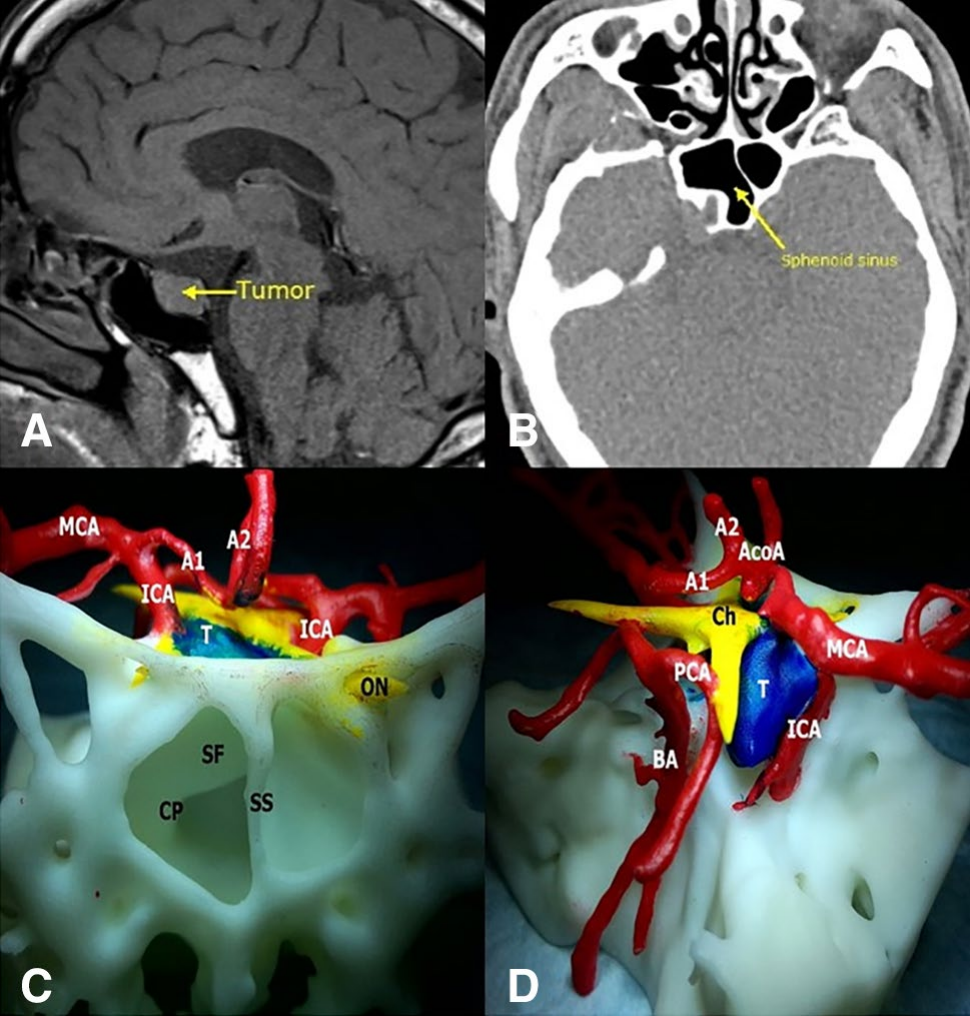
a MRI of the sella turcica region showing tumor lesions. The yellow arrow points to the tumor. b CT of the sphenoidal sinus. The yellow arrow points to the sphenoidal sinus. c, d Frontal and lateral views. *SF* sellar floor, *SS* sphenoid septation, *CP* carotid protuberance, *A1* A1 segment of the anterior cerebral artery, *BA* basilar artery, *PCA* posterior cerebral artery, *A2* A2 segment of the anterior cerebral artery, *Ch* chiasm, *T* tumor, *ON* optic nerve, *AcoA* anterior communicating artery, *MCA* middle cerebral artery
